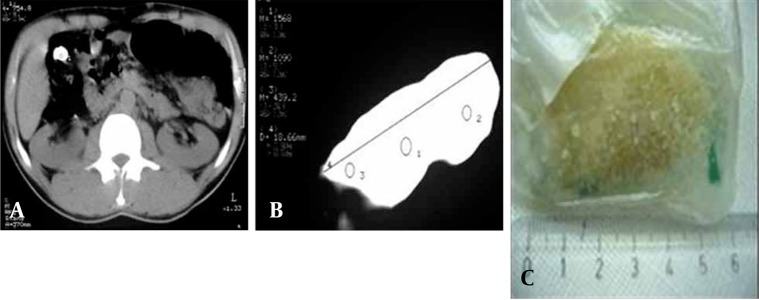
A, Abdominal CT scan without contrast shows a crack packet with the density of more than +1000 HU and length of 6 cm in the hepatic flexure; B, The packet is extracted and evaluated outside the body by CT. The density of the packet is still more than +1000 HU; C, The length of the extracted packet is about 6 centimeters.
